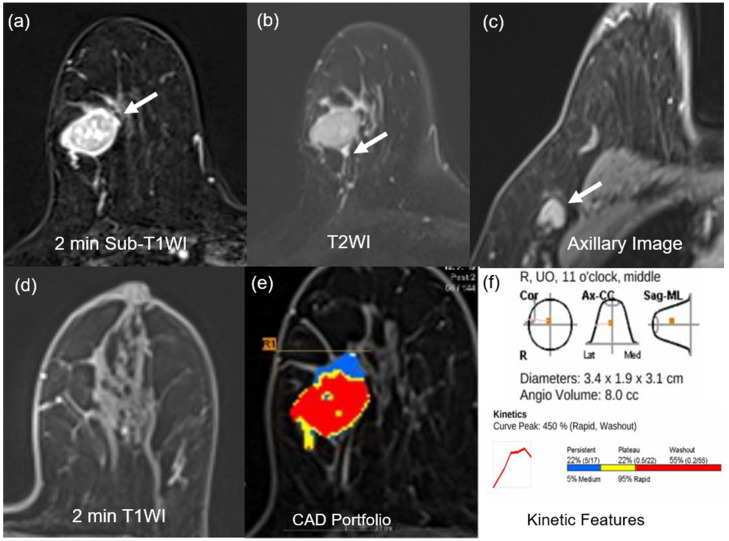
MR images of a 75-year-old woman with a 3.4-cm surgically verified invasive ductal breast cancer and germline BRCA-positivity (a) Axial contrast-enhanced T1-weighted 2 min subtraction image shows an oval, circumscribed, rim-enhancing mass in the right breast (arrow). (b) The T2-weighted image shows peritumoral edema near the mass (arrow). (c) Axillary image shows an axillary adenopathy (arrow). (d) Axial contrast-enhanced T1-weighted 2 min after contrast enhancement image shows minimal background parenchymal enhancement. (e,f) An auto-portfolio of the CAD system demonstrated 3.4 cm in the largest tumor size and 8.0 cc in angio-volume above the enhancement threshold. Delayed-washout component was 55%.
